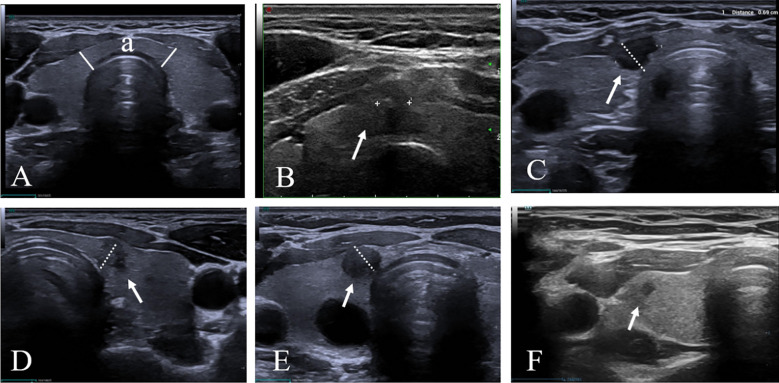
Ultrasound-based definition of isthmic versus non-isthmic thyroid nodules. (A) The anterior contour of the trachea was divided into a curved portion and a relatively straight segment using two solid white lines, defining zone a as the isthmic region. (B, C) Nodules with more than 50% of their cross-sectional area located within zone a were classified as isthmic. (D–F) Nodules located predominantly outside this region were classified as non-isthmic. White arrows indicate the target nodules. White dashed lines represent reference boundaries used to assess whether more than 50% of the nodule lies within zone a.
